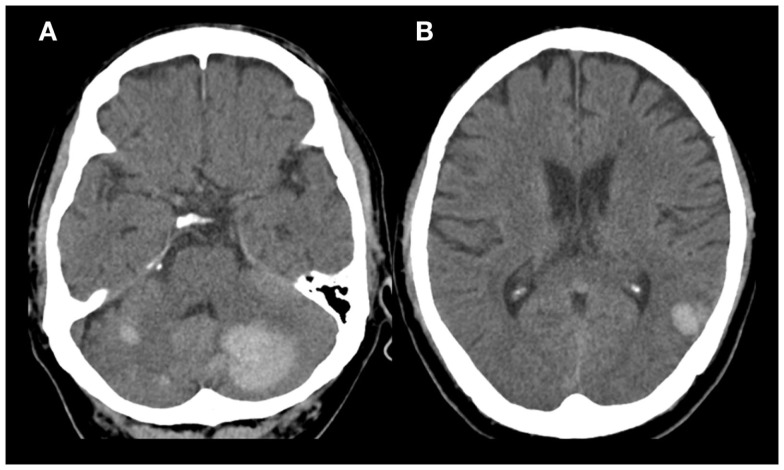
Non-contrast brain CT scan 1 day after thrombolysis. (A) Round shaped (3.1 cm × 3.2 cm × 2.3 cm) hyperdense lesion occupying the left cerebellum and foci of hyperdense lesion in right cerebellum. (B) Foci of hyperdense lesion in left temporal lobe.
